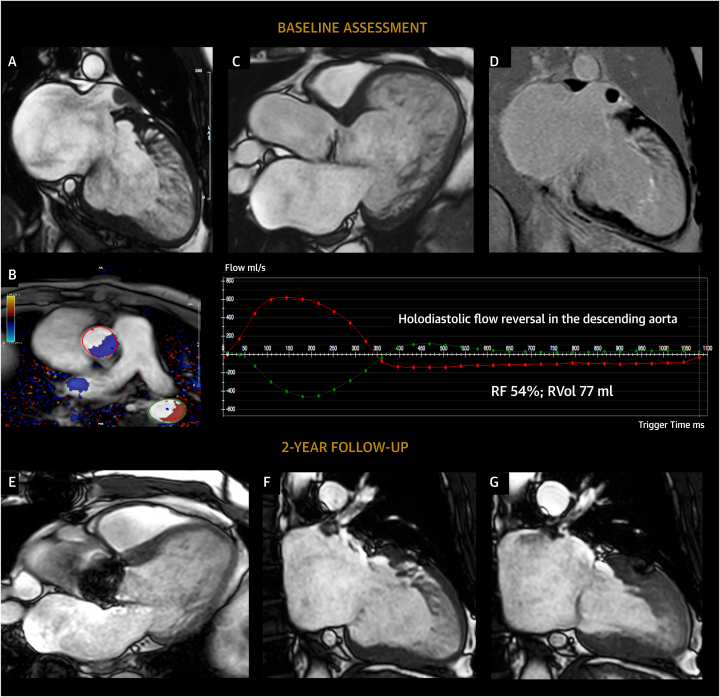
Case Report 4 Images of a 38-year-old master triathlete with history of catheter ablation for persistent atrial fibrillation. Echocardiography at preparticipation screening documented the presence of mildly reduced left ventricular systolic function, mild eccentric aortic regurgitation, and a severely dilated LV with excessive trabeculation (positive Jenni and Chin criteria) that resulted in the diagnosis of so-called left ventricular noncompaction. CMR confirmed the presence of a severely dilated LV with mildly impaired systolic function and excessive trabeculation (A) according to the Petersen criteria (noncompacted [trabecular] to compact layer ratio: 3.1). Severe eccentric aortic regurgitation was present (B and C) in addition to a thrombus within the left atrial appendage (A and D). Six months after surgical aortic valve replacement (E) and left atrial appendage occlusion, significant left ventricular reverse remodeling occurred with near-complete normalization of left ventricular function, volumes, and diameters (end-diastolic frame [F]; end-systolic frame [G]), further leading to a reduction of Petersen criteria for excessive trabeculation (noncompacted [trabecular] to compact layer ratio: 2.4). Moderate-to-severe left ventricular dilatation must be carefully investigated in athletes, irrespective of extent of ventricular trabeculation. If the excessive trabeculation had been part of a cardiomyopathy, arguably, these substantial improvements in left ventricular volumes and function would not have been observed. RF = regurgitant fraction; RVol = regurgitant volume; other abbreviations as in Figures 1 and 4.
